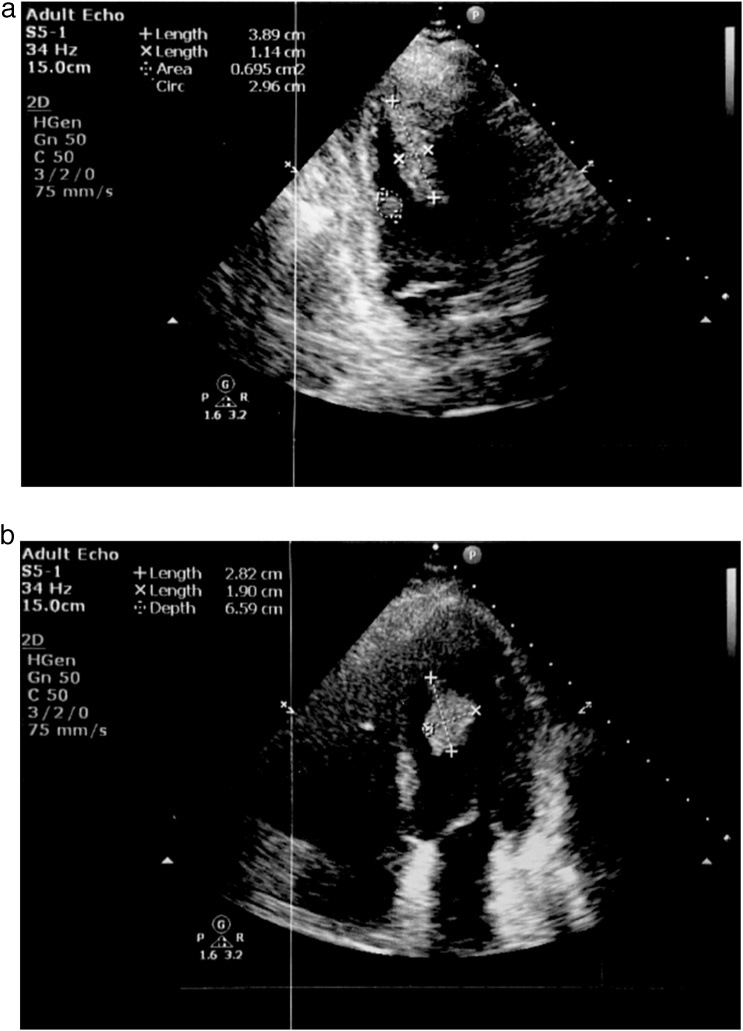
a-b. Echocardiogram of the left ventricle on admission and 10 days after. a Echocardiogram of the left ventricle on admission. b Echocardiogram of the left ventricle 10 days after.
